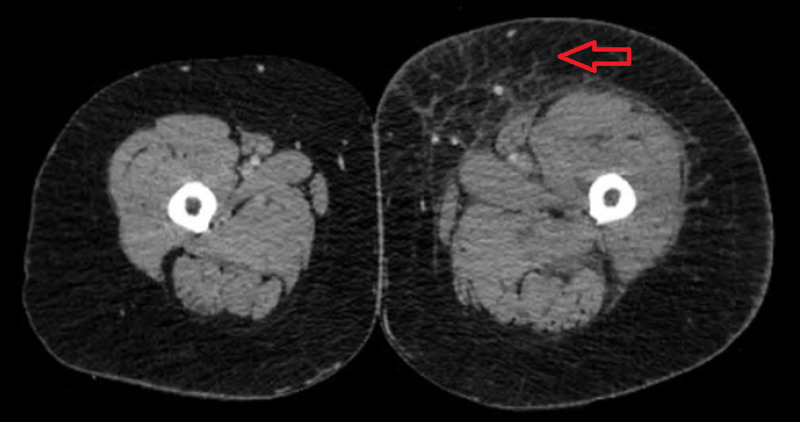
Transverse CT scan of bilateral lower extremities.
